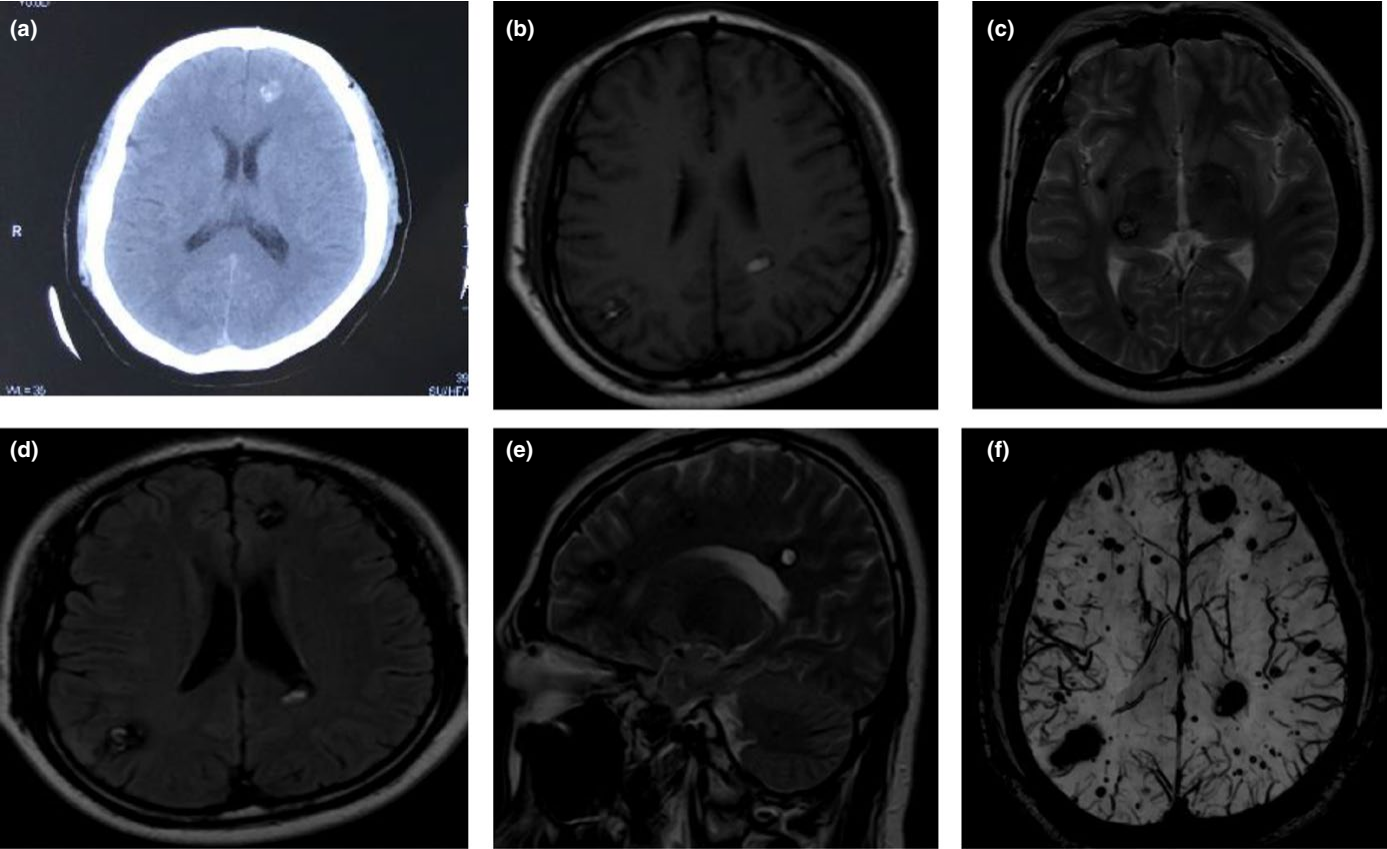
The brain CT and MRI images of the proband's brain is shown. Panel a: CT of the proband. Panel b–e: axial T1, T2‐weighted MRI of the proband's brain; an irregular mixed‐density lesion is shown in the cerebral hemisphere; the hyperdense region represents calcification with bleeding. Panel f: SWI revealed multiple hypointense signals in the cerebral hemisphere of the brain
